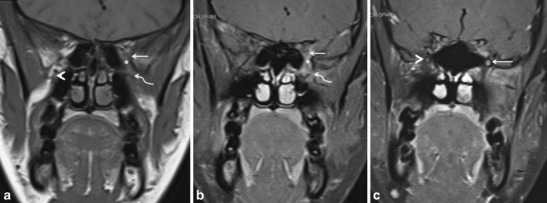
a A 35-year-old female presenting with painful opthalmoplegia of the left eye, diagnosed as IPT. Coronal T1-weighted MR image in the region of the orbital apex shows hypointense infiltrative soft tissue in the left orbital apex (*straight arrow*) extending inferiorly into the left PPF (*curved arrow*) via the left IOF (asterisk). Note the normal hyperintense fat within the right PPF (*arrowhead*). b Corresponding coronal contrast-enhanced FS T1W MR image in the same patient shows enhancing soft tissue in the left orbital apex (*straight arrow*) extending inferiorly into the left PPF (*curved arrow*) via the left IOF (*asterisk*). c Coronal contrast-enhanced FS TIW MR image in the same patient shows enhancement in the enlarged left foramen rotundum (*arrow*) in keeping with retrograde disease spread via the left V2 nerve. Note the normal right foramen rotundum (*arrowhead*)
